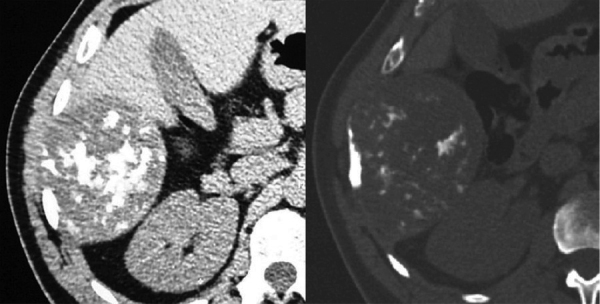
Axial CT scan of a patient with a suspected abdominal mass showing a mass originating in the thoracic wall. Pathological examination demonstrated it to be an enchondroma of the 10th rib.
